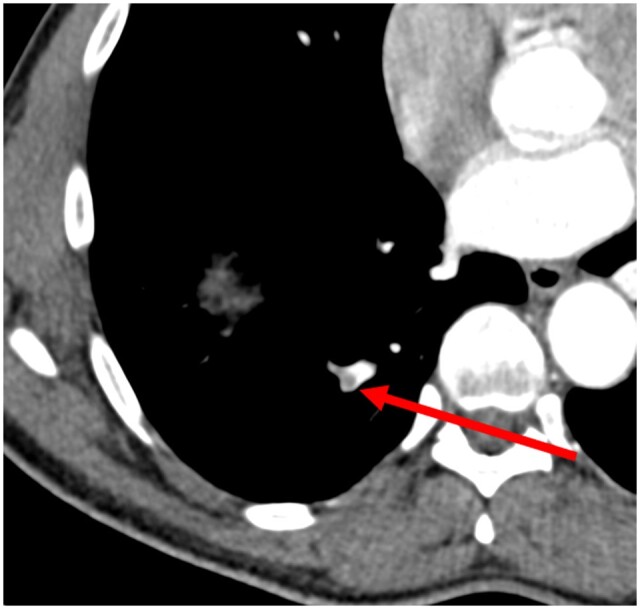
Axial view of missed findings for a pulmonary embolism (arrow) on a contrast-enhanced CT chest.
